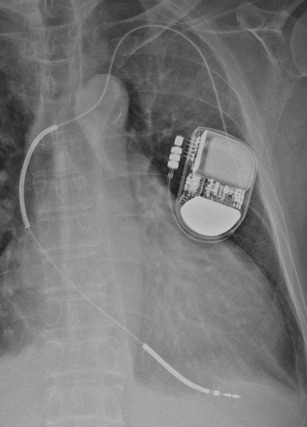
Chest radiograph demonstrating an AICD in situ. A limited number of AICDs are MR-conditional, such as the Medtronic Evera™ MRI ICD and BioTronik ProMRI® AICD
